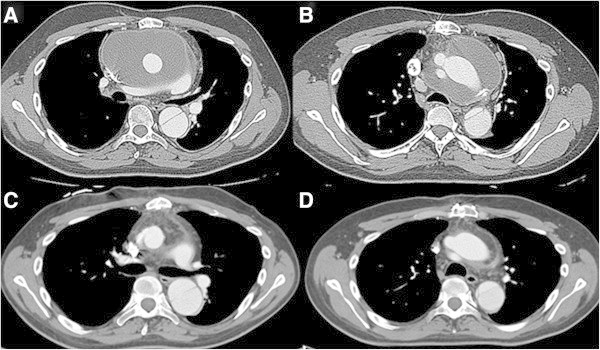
Multi-slice computed tomography (CT) of the chest. Preoperative CT shows a huge mediastinal fluid collection surrounding both A) the ascending, and B) aortic arch prosthetic grafts having a diameter of 15 cm with a low density (12-18 Hounsfield Units). Postoperative CT shows omental wrapping with normal density (65-80 Hounsfield Units) around the C) the ascending, and D) aortic arch prosthetic grafts without evidence of fluid collection or infectious sequela 18 months after the operation.
